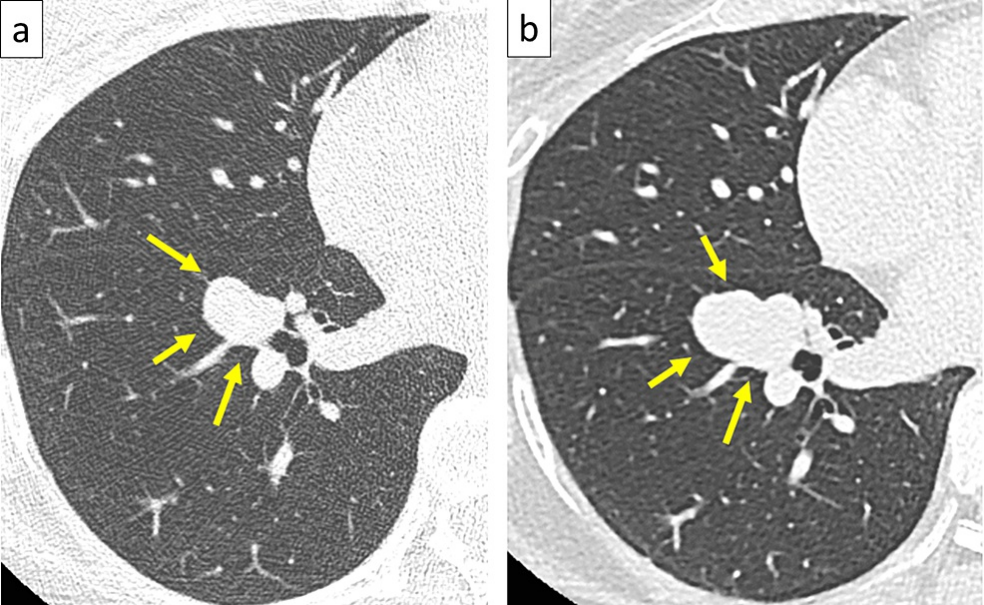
Preoperative imaging findings (a) CT image showing a 15-mm saccular protrusion in the A8 bifurcation of the right pulmonary artery at the time of the initial visit (yellow arrows). (b) Seven years later, a CT image shows the PAA enlarged to 26 mm. CT: computed tomography
